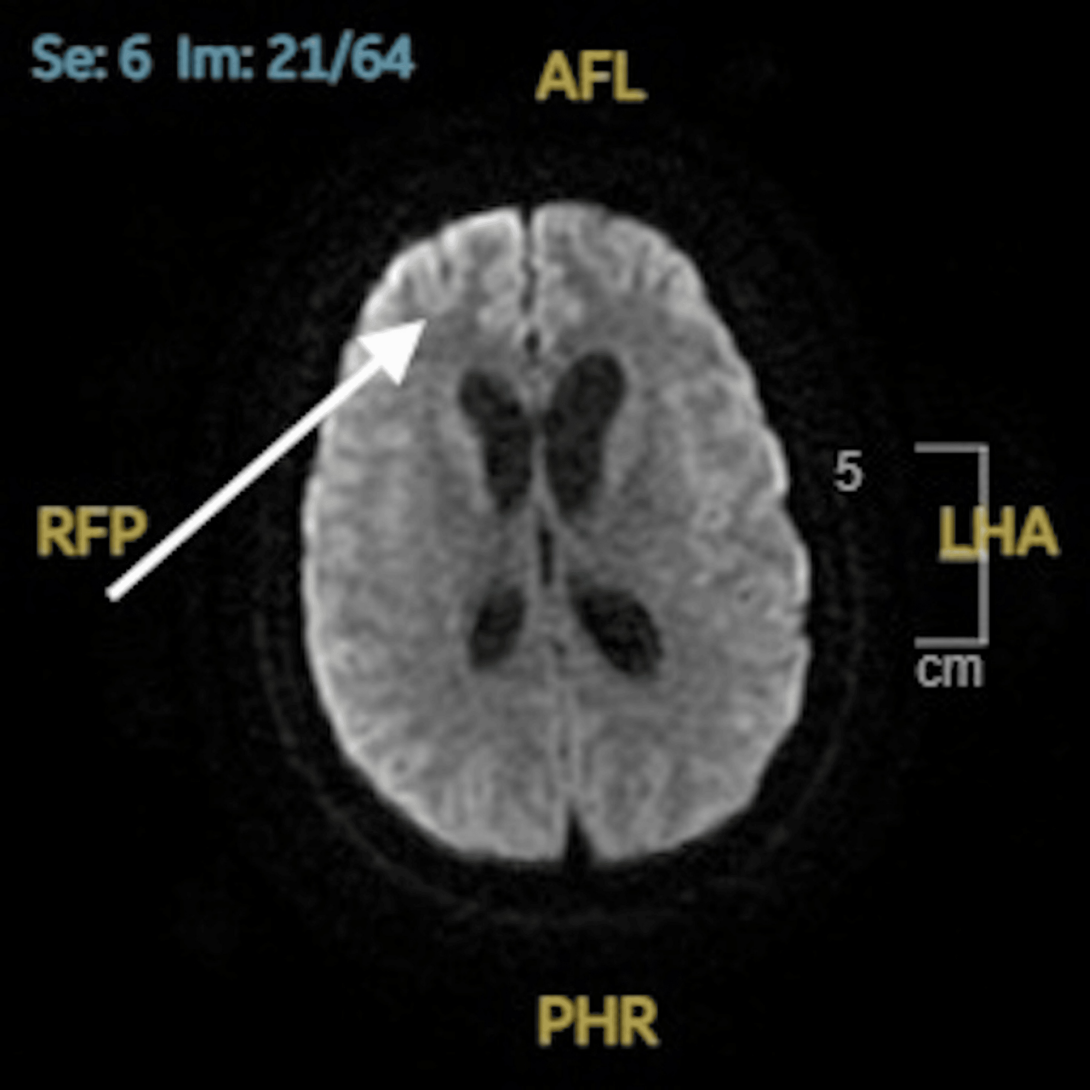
MRI brain axial view with contrast diffusion-weighted demonstrating leptomeningeal enhancement suggestive of meningitis MRI: magnetic resonance imaging; AFL: anterior left; LHA: left anterior; PHR: posterior right; RFP: right posterior
